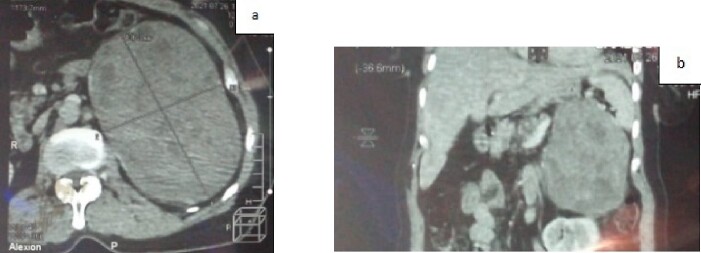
a. CT scan of the abdomen showing a voluminous left adrenal mass (axial section); b. CT scan of the abdomen showing a voluminous left adrenal mass (coronal section).
